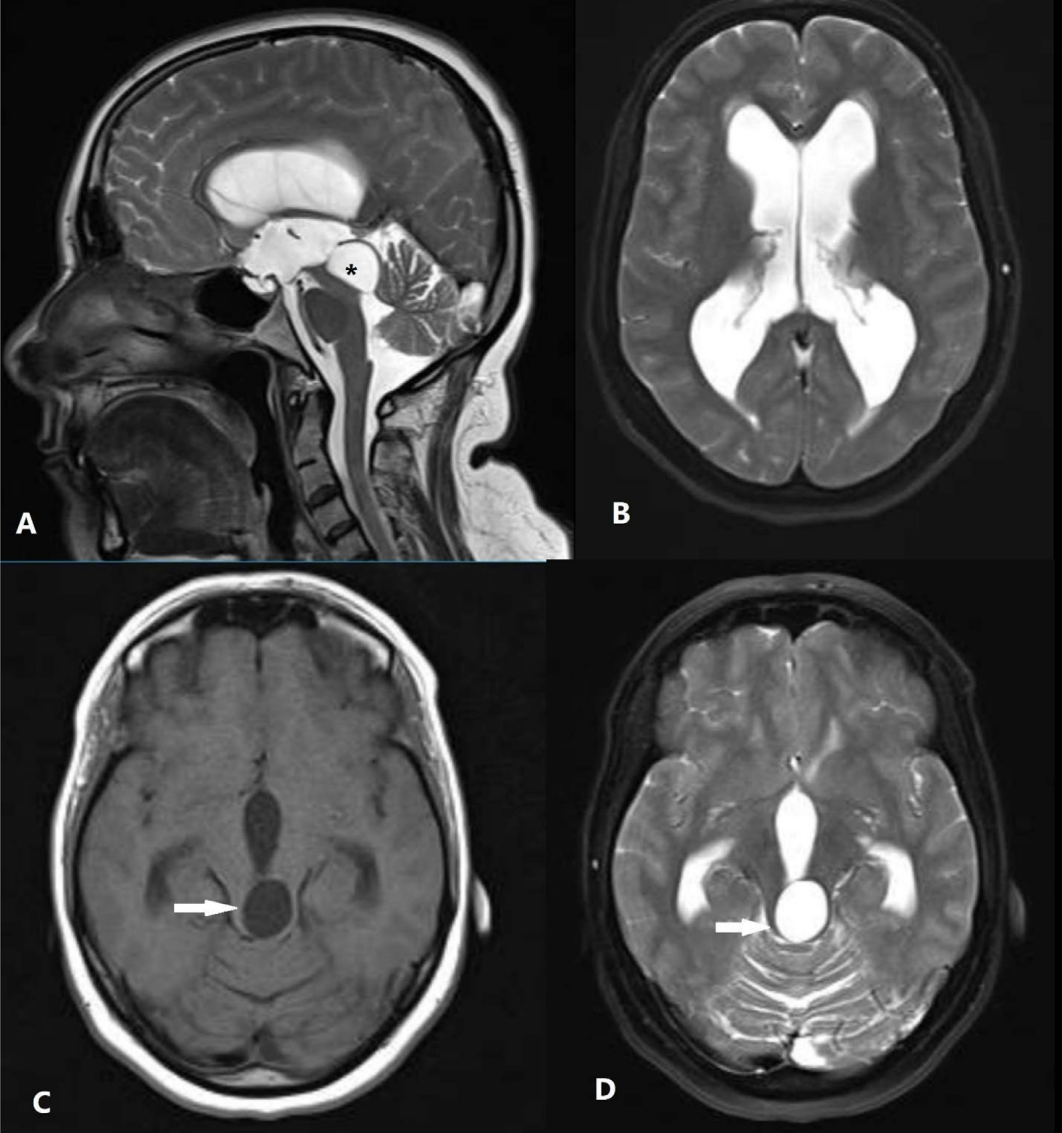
Sagittal and the axial plane of the brain magnetic resonance imaging T2/FLAIR (A, B) sequences: There is a 21*18*20 mm cyst from a tectal plate covered by a thin rim of tectal plate tissue following cerebrospinal fluid (CSF) signal (black asterisk). The axial plane of the brain T1 (C) T2 (D) sequences: The cyst was homogeneously low on T1 and high on T2 sequences (solid white arrows).
